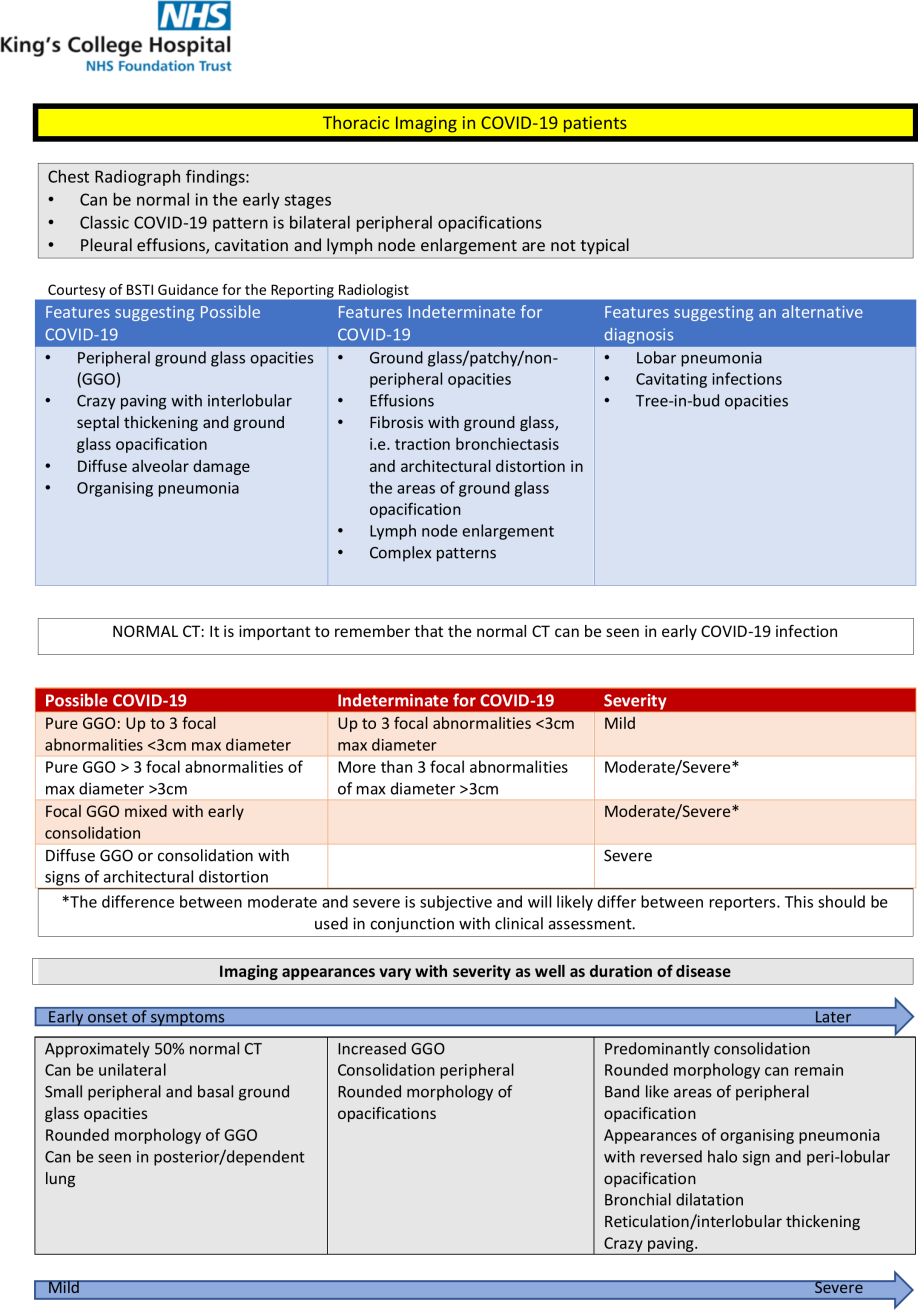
Departmental guidelines for the identification and reporting of COVID-19 on CT Chest. Courtesy of King’s College Hospital NHS Foundation Trust.
